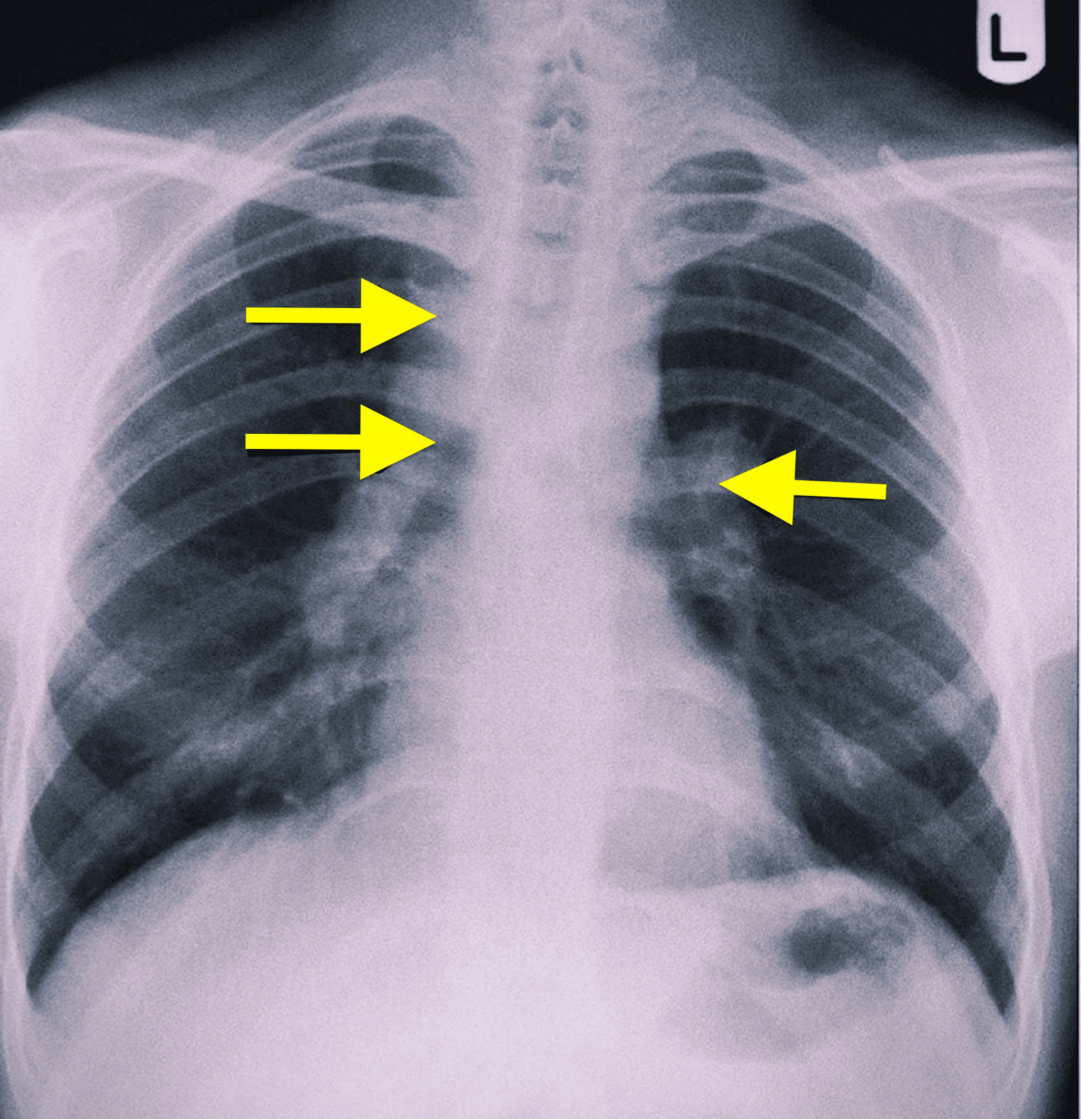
Chest radiograph posteroanterior view showing bilateral hilar and right paratracheal adenopathy
